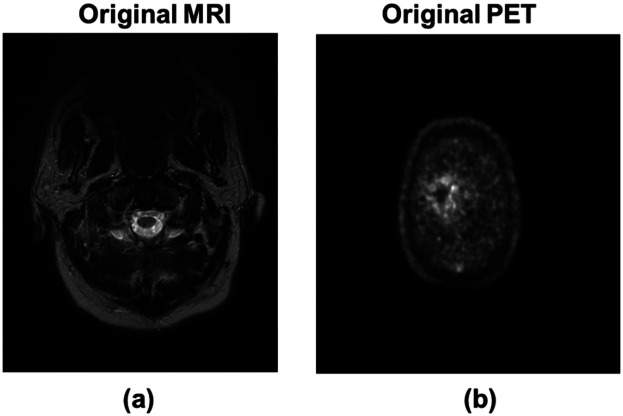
Example of (a) MRI and (b) PET data images from Kaggle.
